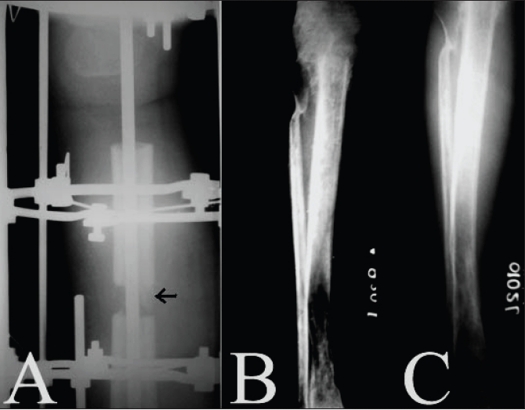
A) X-ray after resection of GCT of pxoximal tibia and distal tibial corticotomy. The tibia has been fixed with an intramedulary nail and ilizarov external fixator. Arrow shows regenerate at the corticotmy site. B) Follow up X-ray showing complete docking at the resection site and regenerate at the corticotomy site. C) X-ray shows union at the resection site and consolidation at the corticotomy site.
